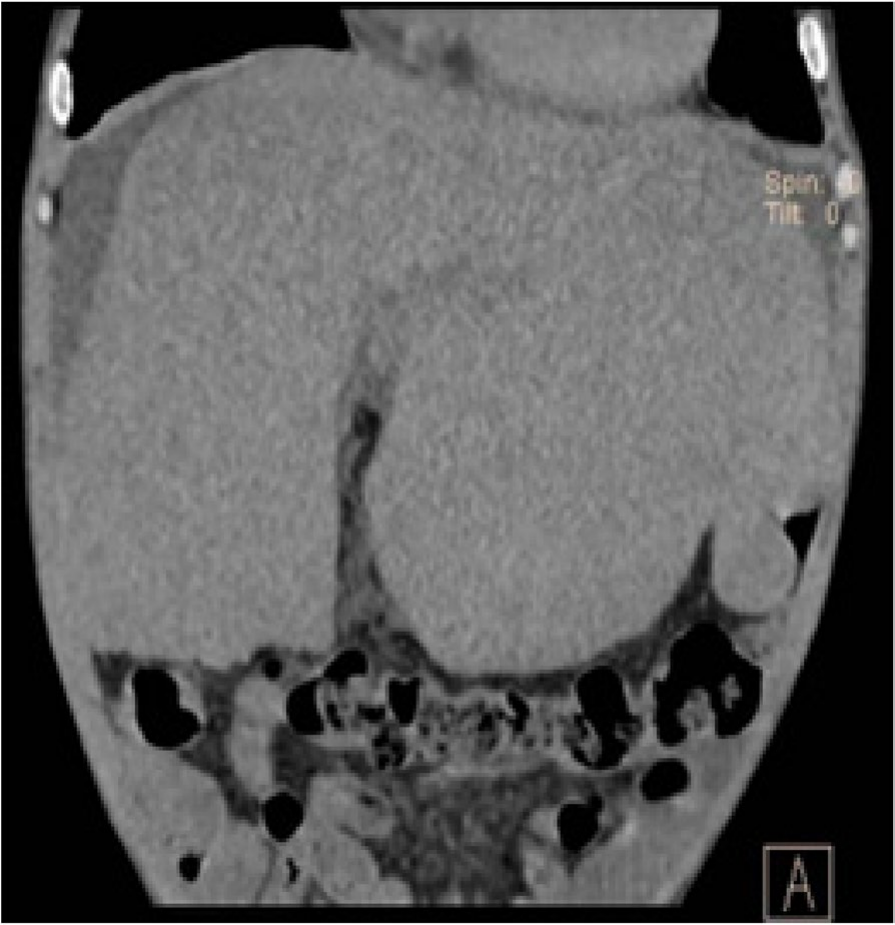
Abdominal CT showed persistent enlarged liver but few ascites after 8-week follow-up. CT=Computed tomography
